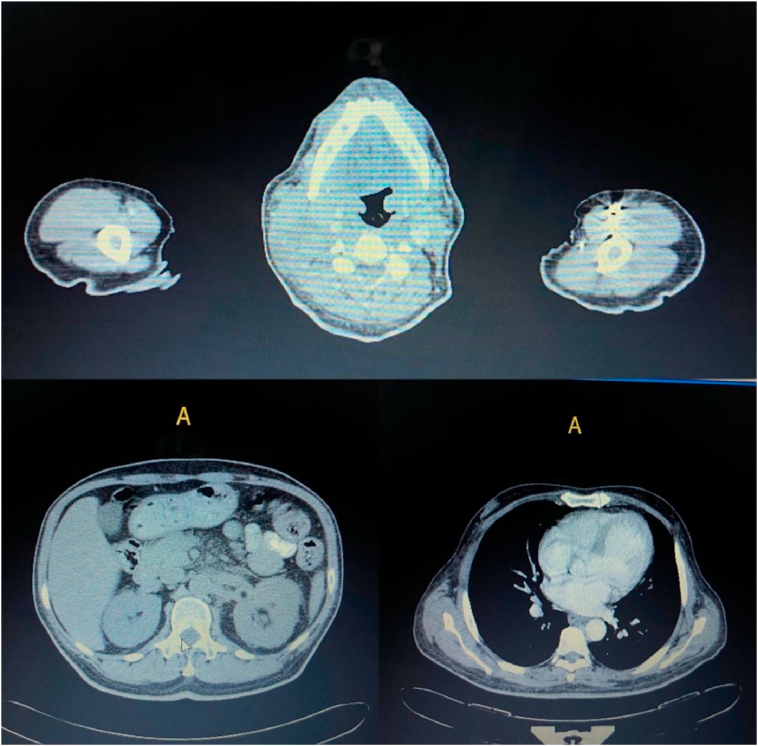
CT scan showing a conglomerate of enlarged pathological lymph nodes, some with necrotic centers, in the right cervical region, right and left side of the chest and peri-aortic region.
